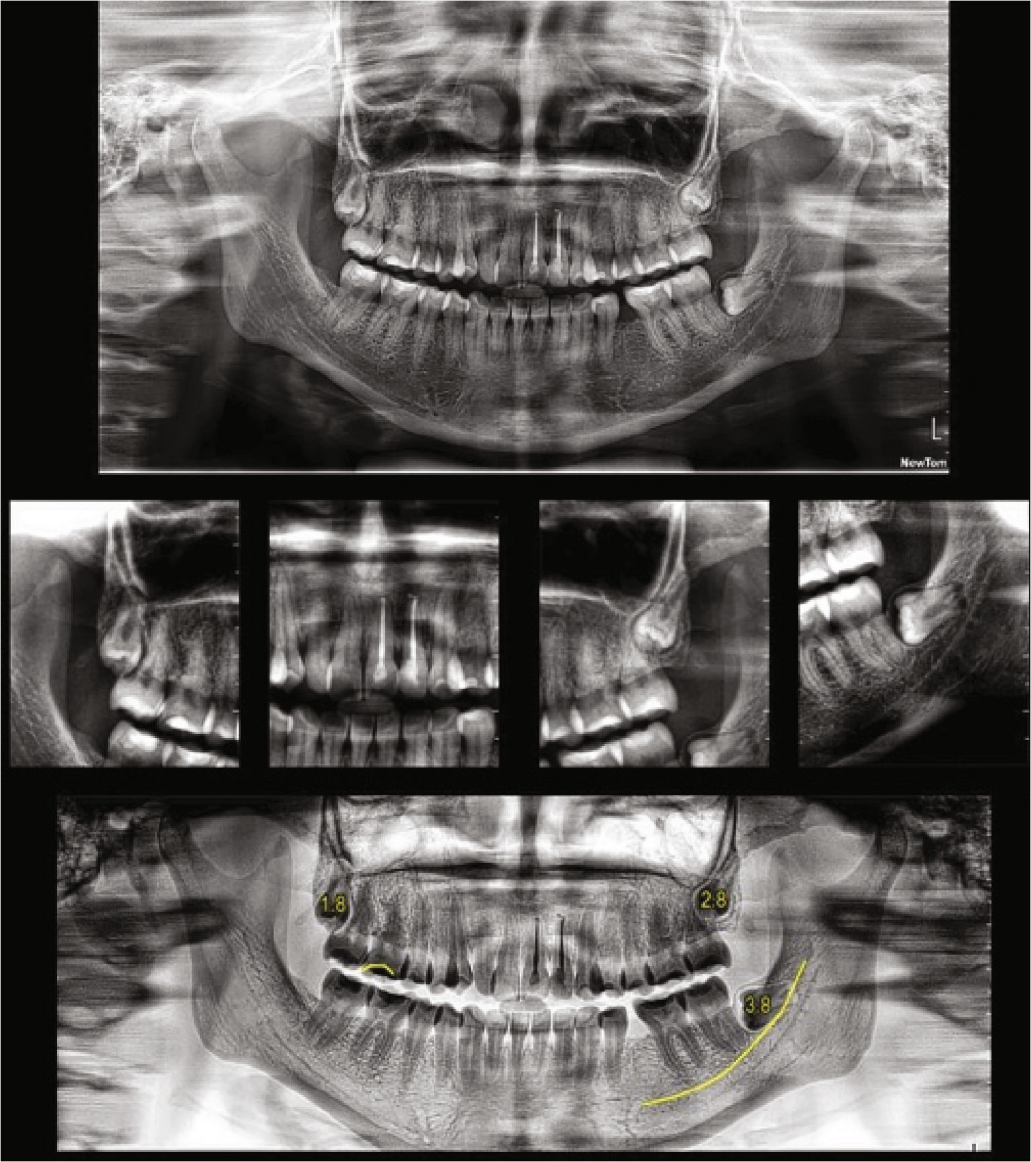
Annual radiographic control.
